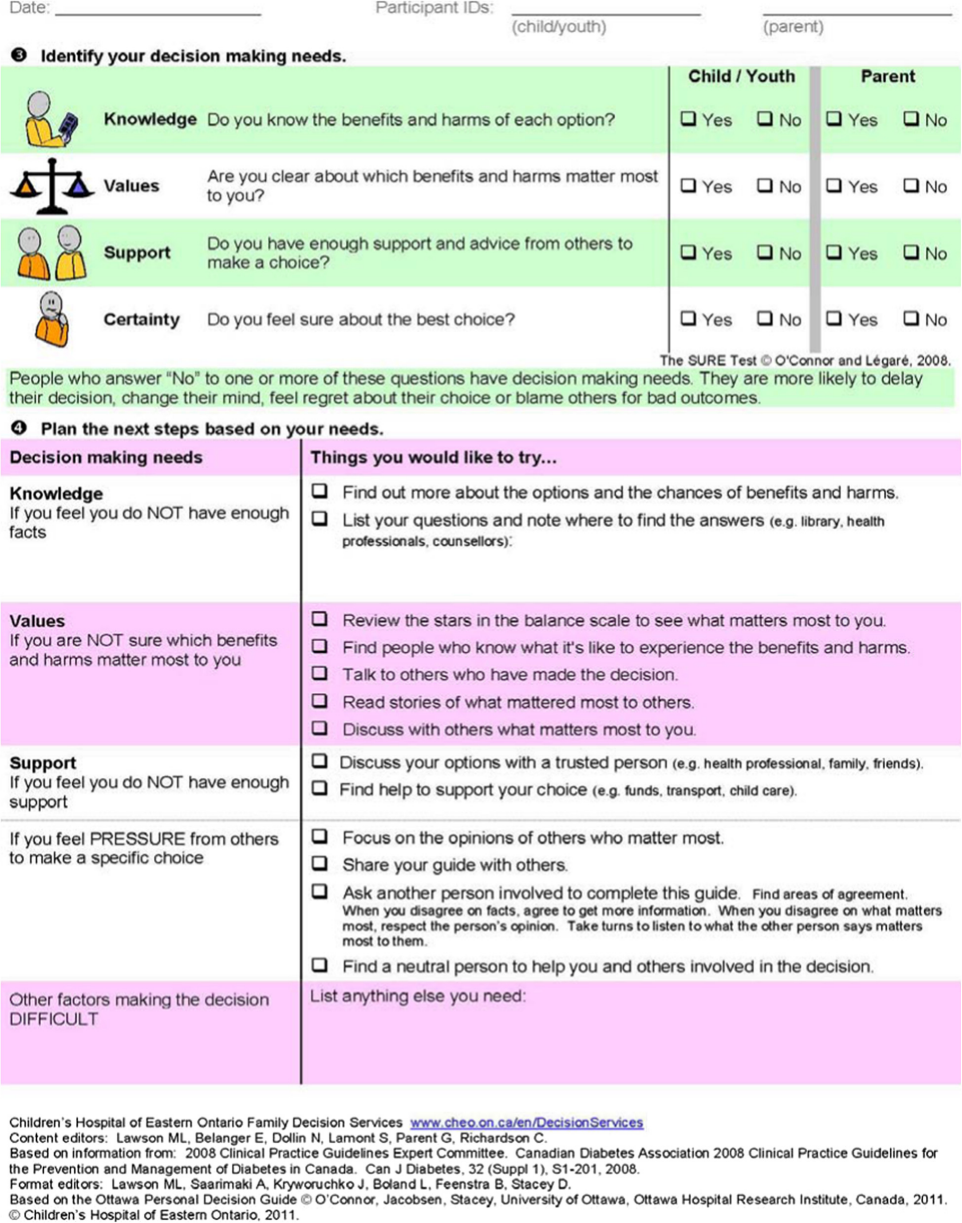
OFDG pre-populated with insulin options for type 1 diabetes management (page 2).
